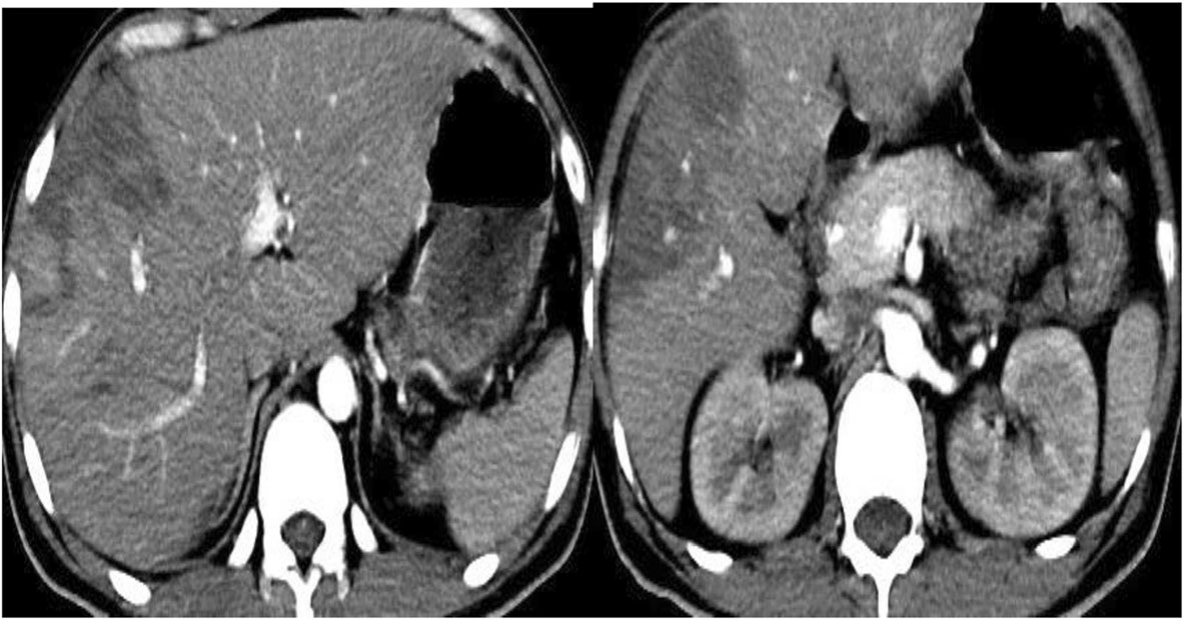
Abdominal CT showing multiple focal lesions in the liver
